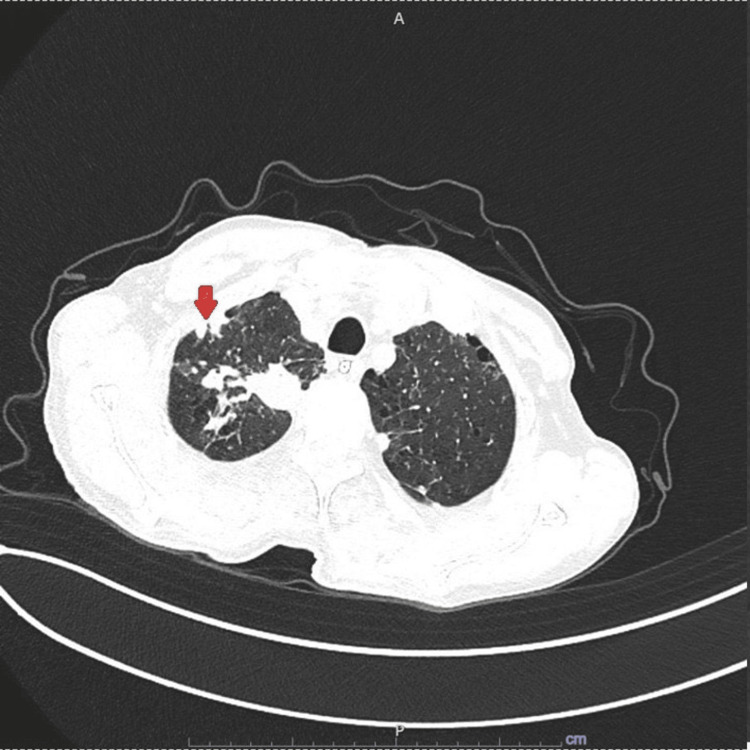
CT of the thorax (axial): thick-walled cavity with surrounding consolidation (red arrow)
